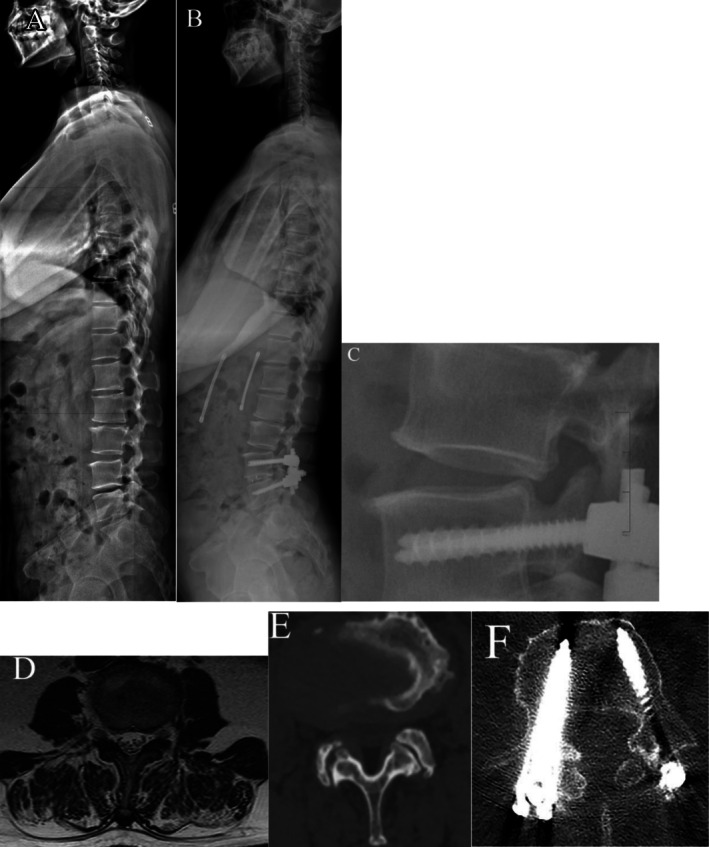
(A) A 47‐year‐old female patient diagnosed with L4/5 lumbar spinal stenosis who subsequently underwent L4/5 TLIF. (B, C) Standing lateral radiograph at 5‐year follow‐up demonstrates L3 retrolisthesis, accompanied by new‐onset lower limb symptoms. (D) Preoperative axial and sagittal T2‐weighted MRI at the L3/4 level reveal marked fatty infiltration of paraspinal muscle. (E, F) Preoperative CT demonstrates Grade 3 facet joint degeneration at L3/4, and postoperative CT confirms Grade 3 facet joint violation at the same level.
